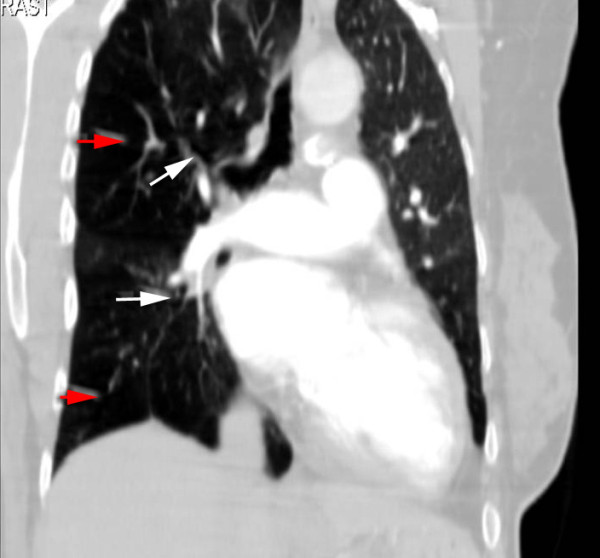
Chest angio-computed tomography revealing hypoplasia/agenesis of the right pulmonary artery (white arrows) and hyperinflation of the pulmonary parenchyma (red arrows).
